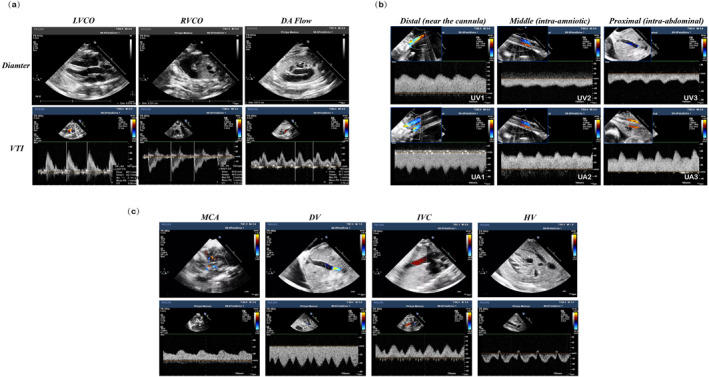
Ultrasonic parameters obtained in fetal lambs on EXTEND support. (a) Measurement of diameters and spectral Doppler waveforms of aortic valve, pulmonary valve, and ductus arteriosus. (b) Spectral Doppler waveforms and measuring sites of the distal (near the cannula tip, UV1/UA1), middle (intra‐amniotic, UV2/UA2), and proximal (intra‐abdominal, UV3/UA3) portions of umbilical artery and vein. (c) Spectral Doppler waveforms and measuring sites of middle cerebral artery (MCA), ductus venosus (DV), inferior vena cava (IVC), and hepatic vein (HV).
